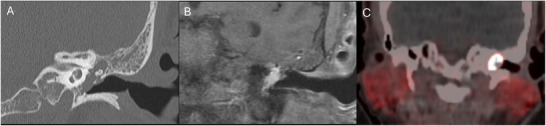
Multi‐modal imaging of a glomus tympanicum with positron emission tomography/computed tomography (PET/CT) and magnetic resonance imaging (MRI). Imaging of a left glomus tympanicum, a benign paraganglioma of the middle ear, which is highly vascularized. The mass completely opacifies the middle ear cavity on coronal CT (A), with avid enhancement on MR (B) and 68‐gallium dotatate hypermetabolism on PET/CT (C).
